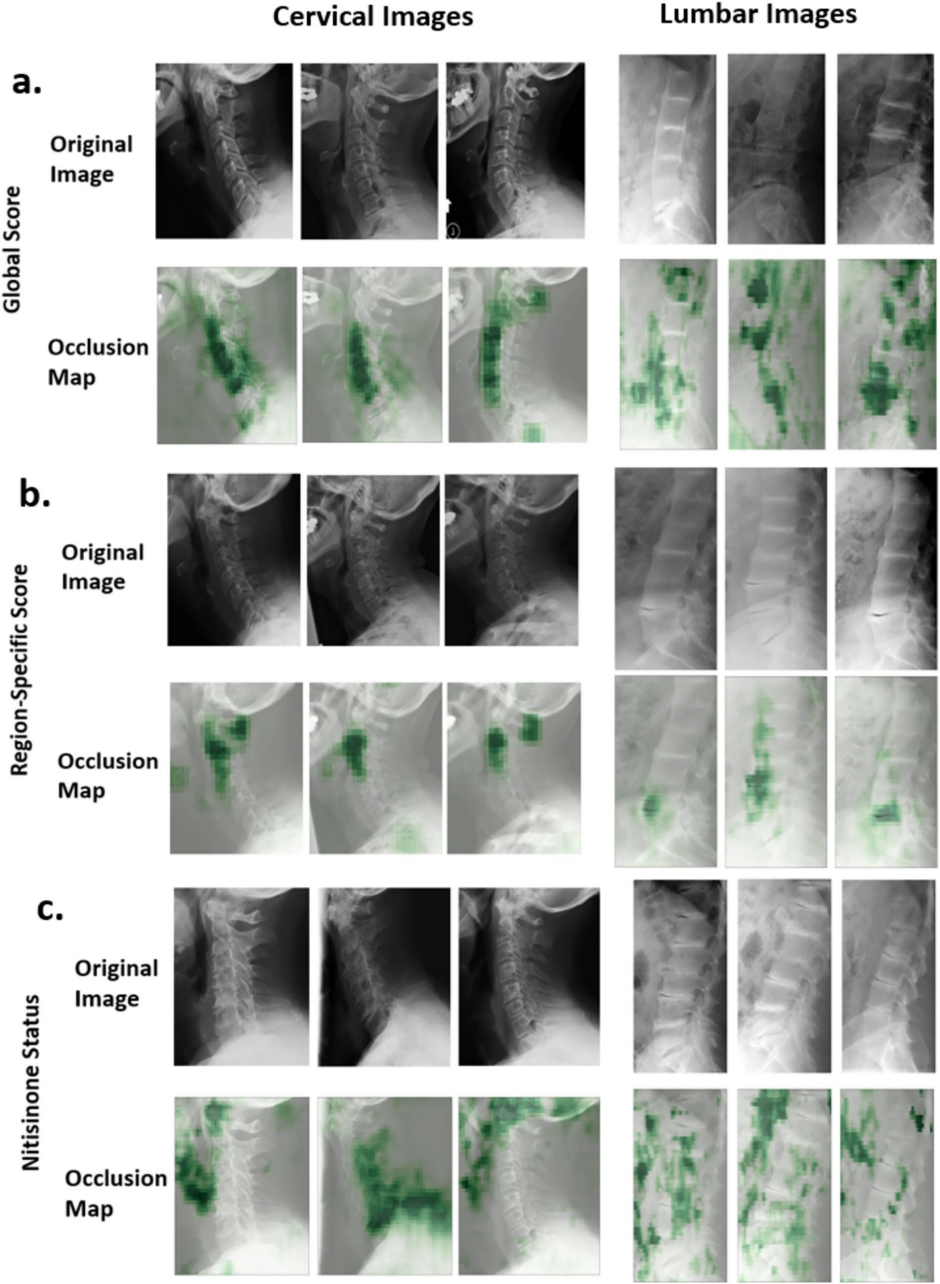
Examples of saliency maps for model prediction of (a) global scores, (b) region‐specific scores, and (c) nitisinone status on cervical and lumbar AKU radiographs. Green, shaded regions indicate regions of model attention when predicting each metric for the entire cervical or lumbar image.
